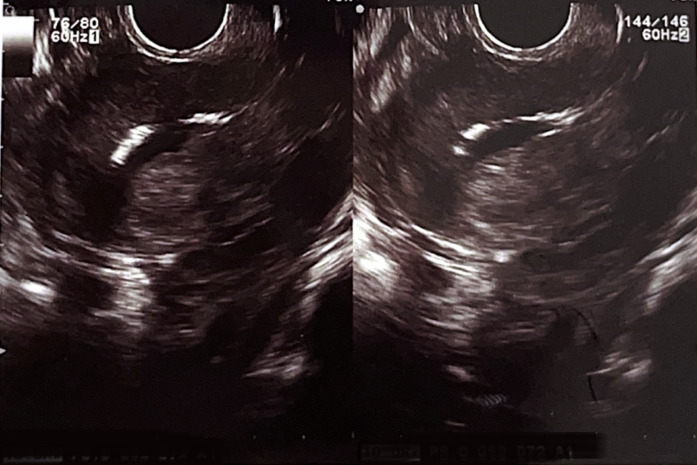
saline infusion sonography showing dense linear hyperechoic substances in the endometrium with acoustic shadowing
